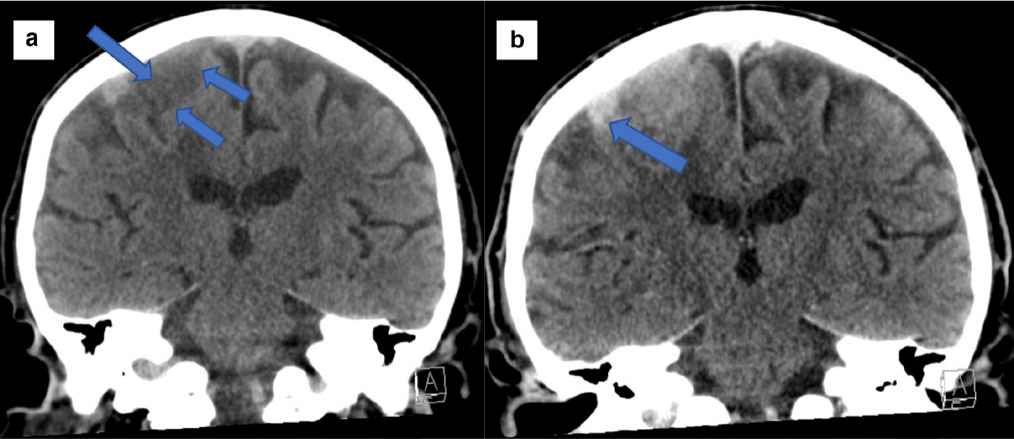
(A) Pre-Contrast Coronal CT Head demonstrating a 34 × 32 × 23 mm area of low attenuation soft-tissue extradural mass (Blue Arrow), which is overlying the superior right parietal lobe at the vertex. There is also a moderate degree of mass effect exerted upon the underlying cortex with sulcal effacement. (B) Post Contrast Coronal CT Head demonstrates relatively high-attenuation material (75 H.U.) within the lesion (Blue Arrow)
